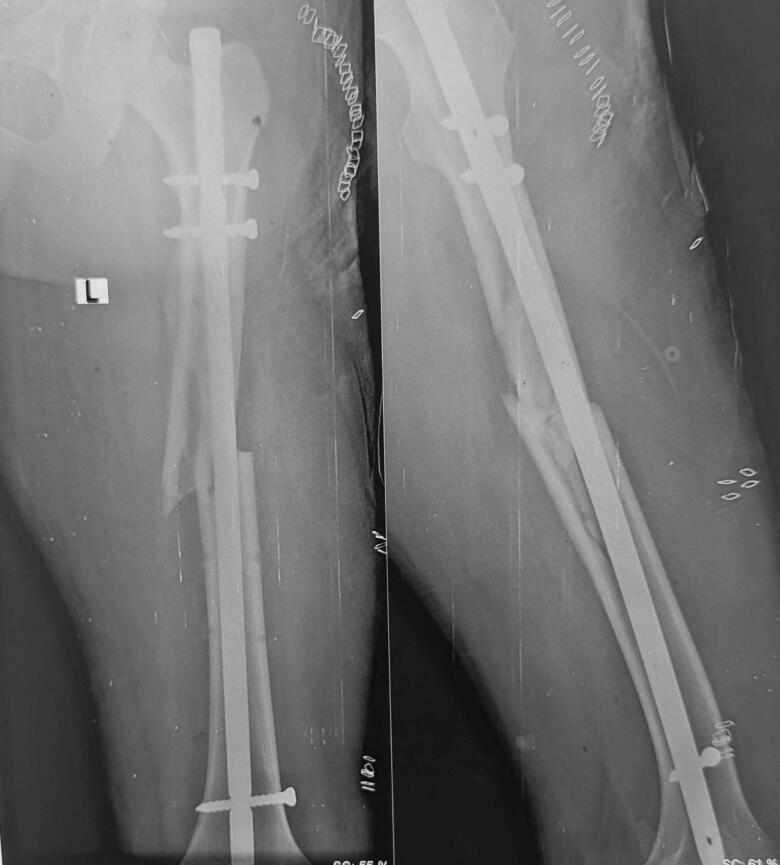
Post-operative x-ray left femur full length anteroposterior and lateral view shows intramedullary femoral nail in situ.
